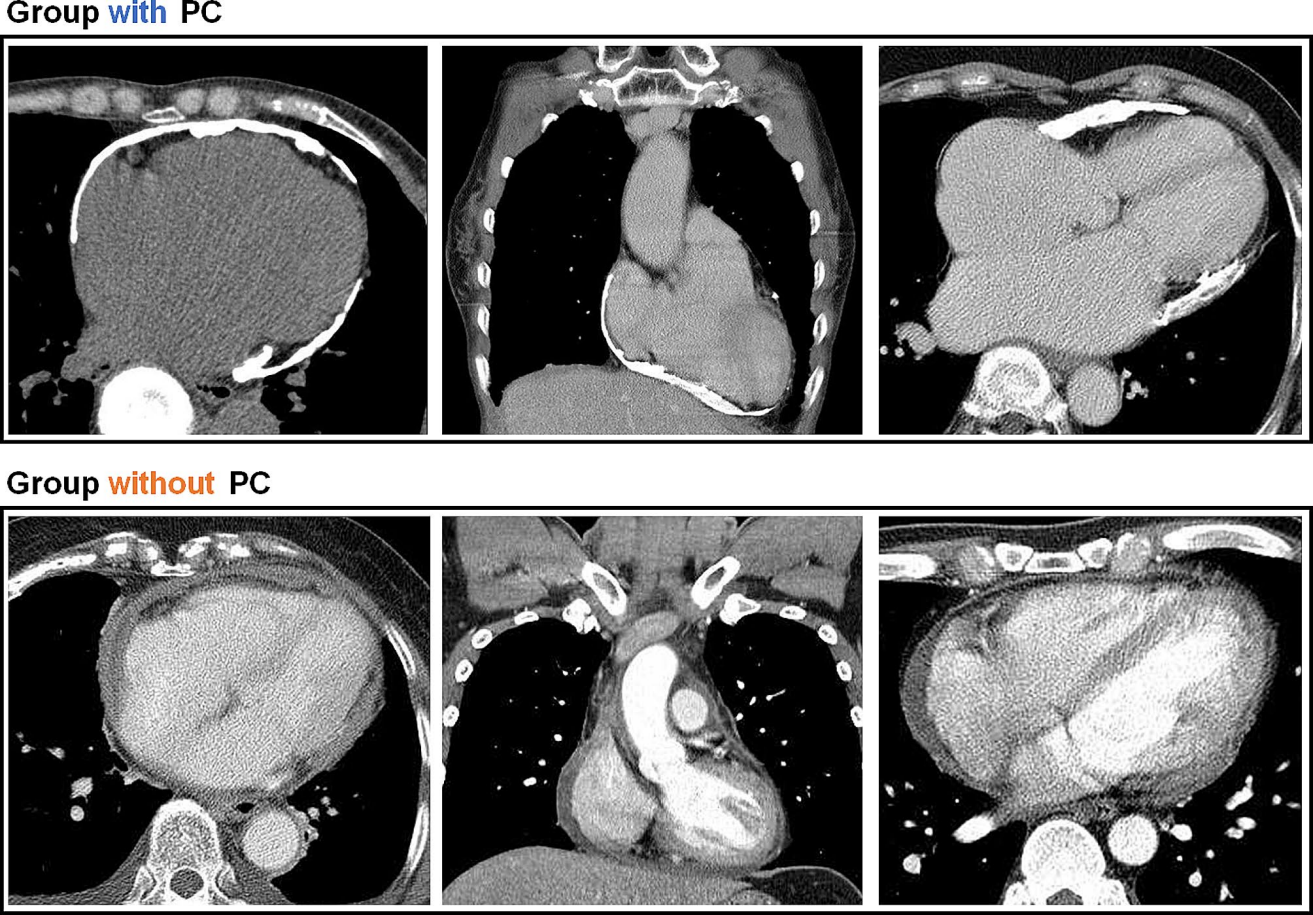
Comparative preoperative CT Imaging of patients with constrictive pericarditis, highlighting cases by presence or absence of pericardial calcification. CT, computed tomography; PC, pericardial calcification
